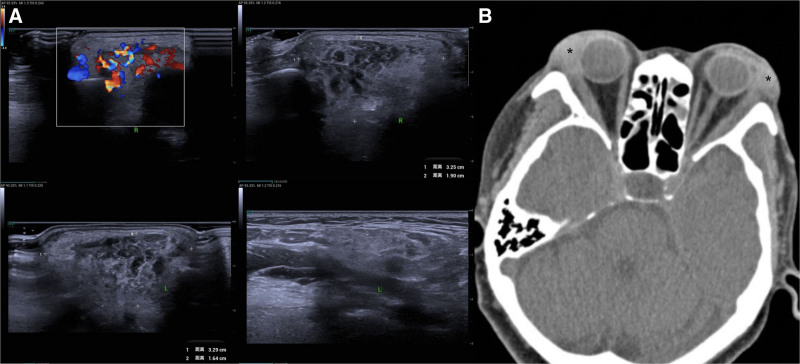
Imaging data. Color Doppler ultrasound reveals bilateral lacrimal glands that are plump, thickened, and diffusely enlarged, with the right measuring approximately 3.25 × 1.90 × 1.93 cm and the left approximately 3.29 × 1.64 × 2.12 cm. The glands exhibit relatively regular morphology with slightly ill-defined margins. Their internal echotexture is heterogeneous, showing hypoechoic to isoechoic signals interspersed with patchy hypoechoic areas and a reticular pattern. Marked hypervascularity is demonstrated, characterized by abundant blood perfusion (Panel A). Orbital computed tomography (CT) shows diffuse soft tissue edema in the superolateral orbital lacrimal gland region. The lacrimal gland is diffusely enlarged, with irregular morphology and ill-defined borders. Plain scan reveals uniform slight hyperdensity (mean Hounsfield unit ≈ 100, *marked), without definite hypodense lesions or calcific foci in the glandular parenchyma. Orbital wall bony structures are intact, with no destruction or proliferative changes (Panel B).
